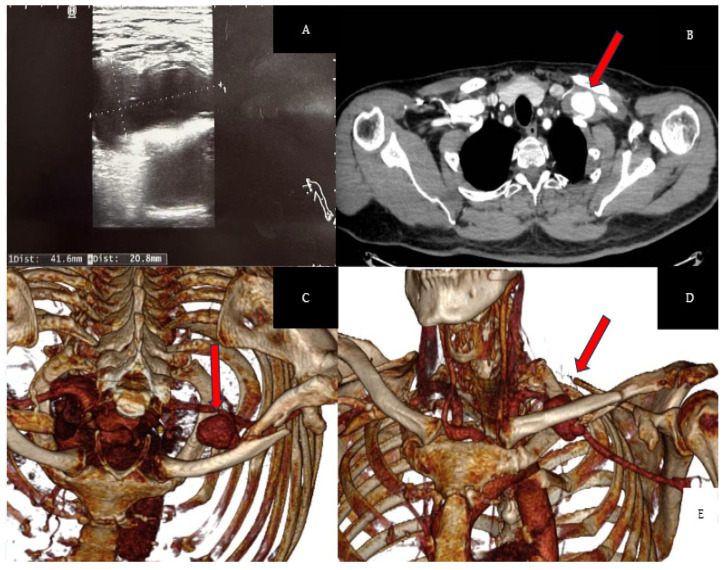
(A) Doppler ultrasonography indicated pseudoaneurysm. (B) Chest CT scan (axial plane) confirmed subclavian pseudoaneurysm. (C,D) 3-dimensional image showing the pseudoaneurysm (red arrows).
